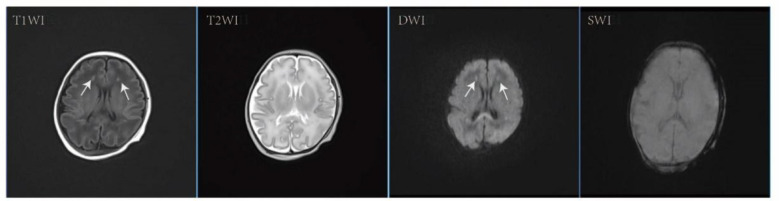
MRI images of punctate white matter injuries. T1WI and DWI showed increased signal intensity near the anterior horn of the lateral ventricle. T2WI showed decreased or loss of signal intensity in the same localization.
